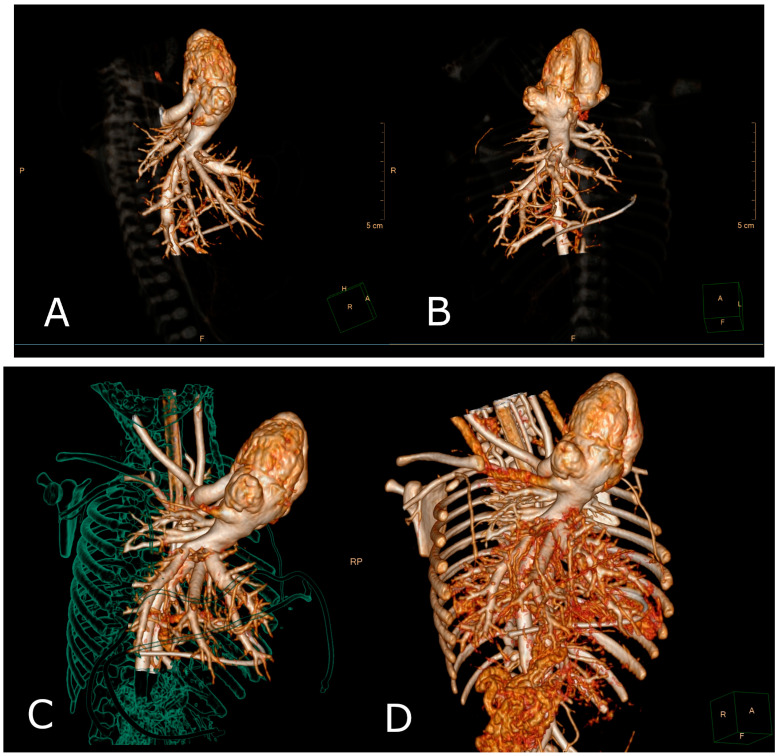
A three-dimensional computed tomography angiography (CT 3D) image revealed the entire heart external to the thoracic cavity with the apex oriented superiorly to the left-lateral view (A) and anterior view (B). (C) The 3D-volume rendering of the thoracic cavity with relation to vital organs and the lack of anterior coaptation of the chest wall components. (D) This image demonstrated mediastinal vascular malformations surrounded by thoracic vertebrae, short, hypoplastic ribs and sternal cleft.
